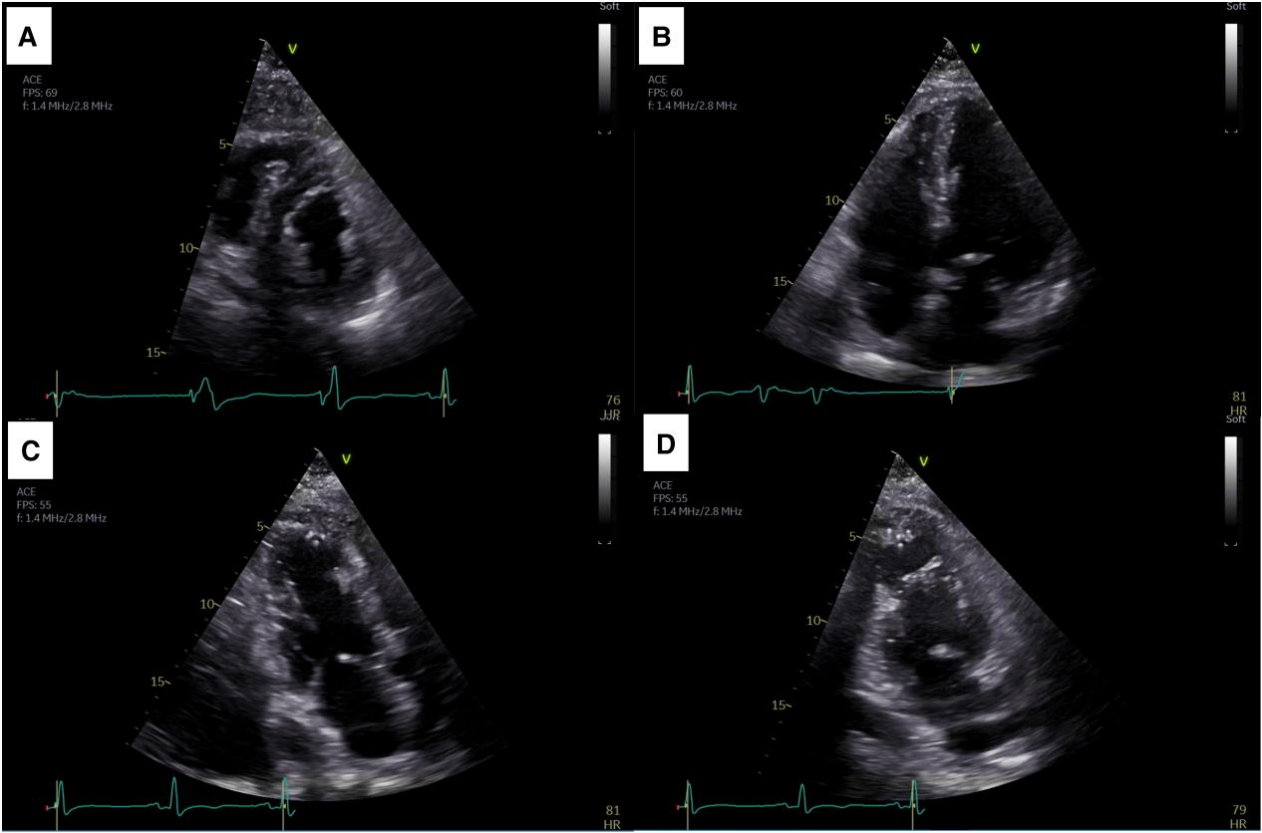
Transthoracic echocardiogram after surgery exhibiting the absence of residual ventricular septal rupture or left ventricular thrombus, and complete recovery of left ventricular ejection fraction to 55%, despite apical and mid-wall interventricular septum segment akinesia. (*A*) Parasternal short-axis view at the apical level. (*B*) Apical four-chamber view. (*C*) Apical three-chamber view. (*D*) Apical two-chamber view.
